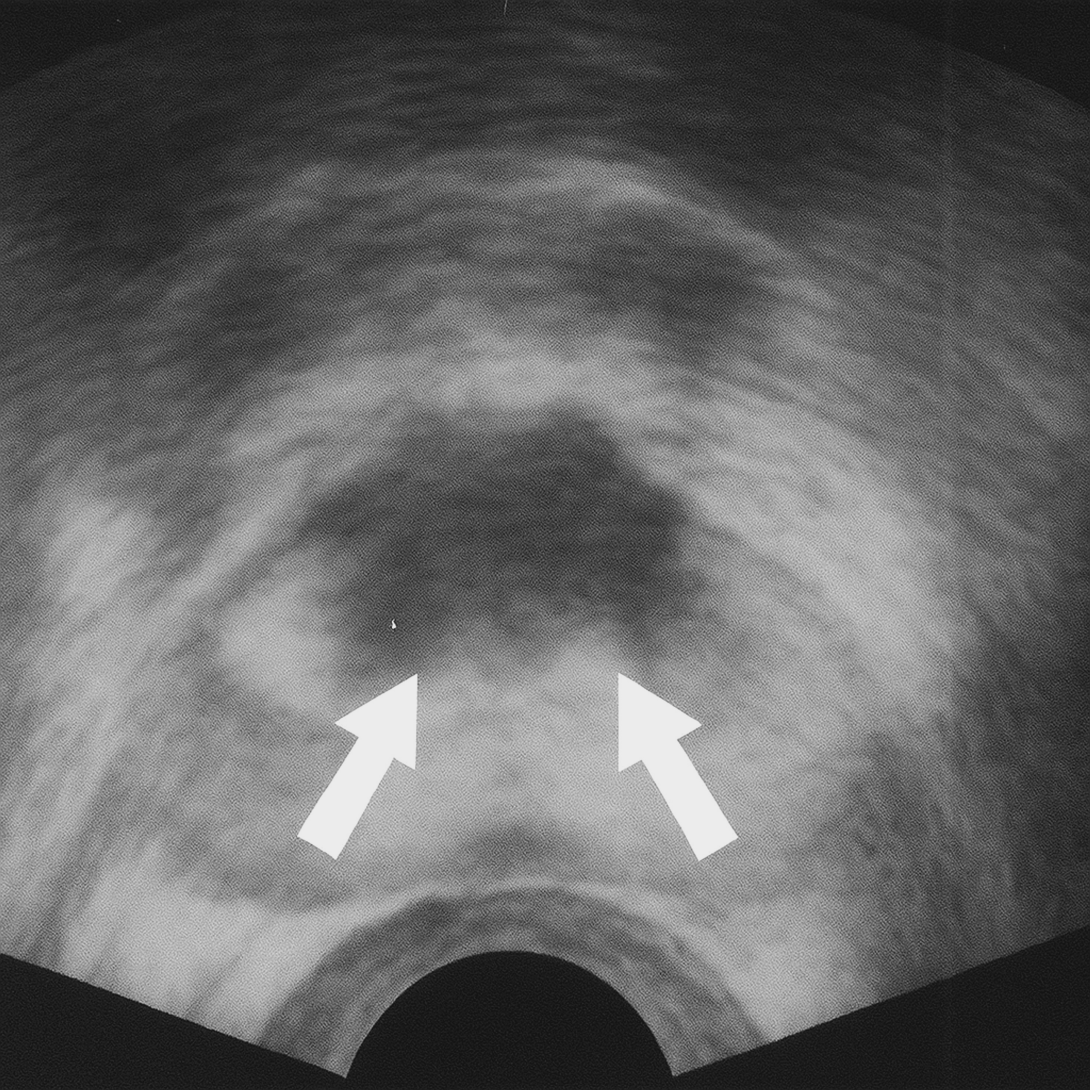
TRUS before resection (sagittal). Hyperechoic focus with posterior acoustic shadowing corresponding to the calcification (digital arrows point to the calcification/shadowing border) mapped on preoperative CT (original image). Source: Authors; no copyrighted material used; permission not required. TRUS: Transrectal ultrasound
